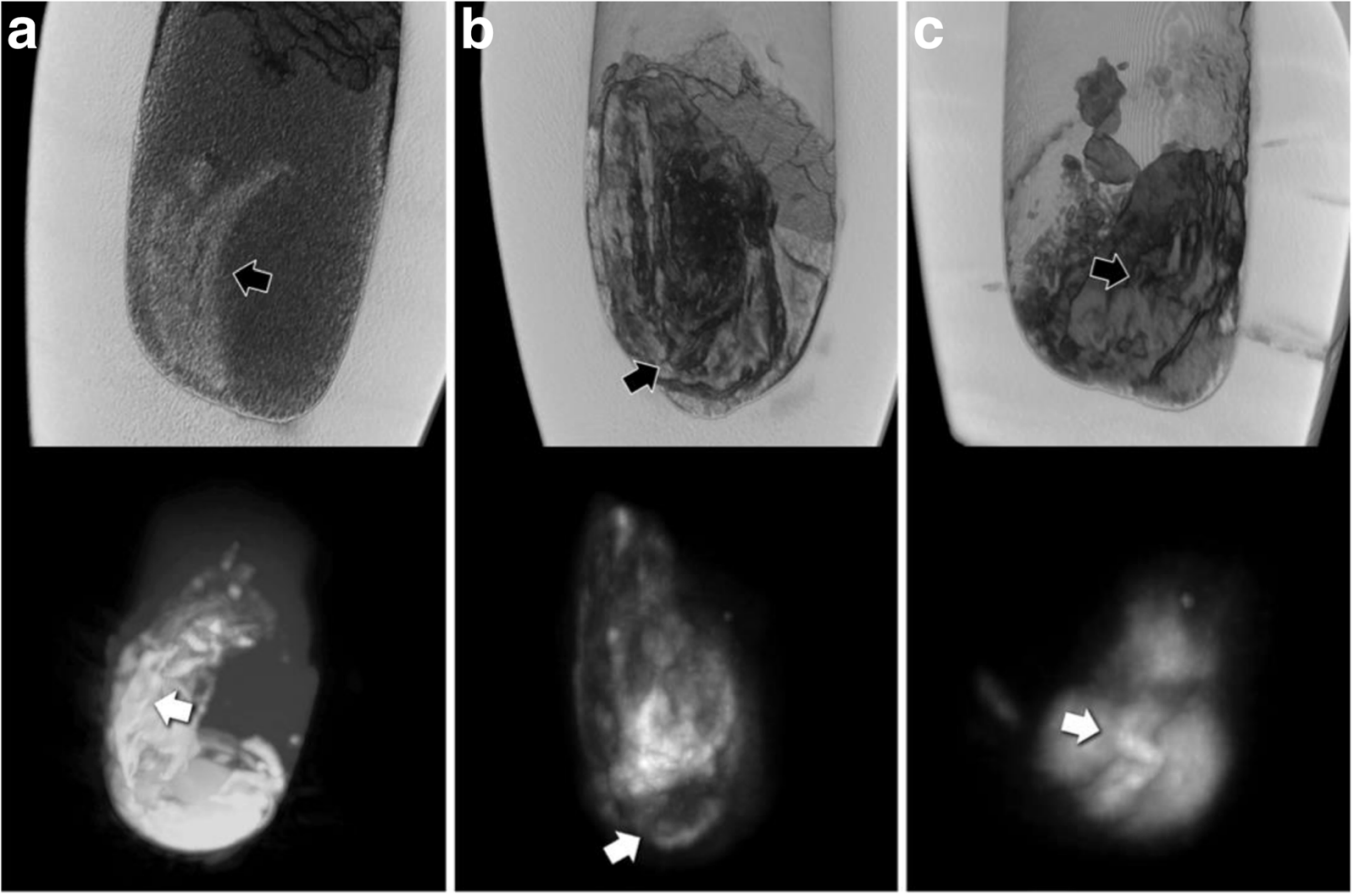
CT reconstructions (*top*) and correlative maximum intensity projections of MRI scans (*bottom*) of the three canopic jars with solid contents (a, inventory number 607; b, inventory number 617; c, inventory number 622-1). *Black arrows* (on CT) and *white arrows* (on MRI) indicate structures of organ-fragment-like morphology, probably intestine
